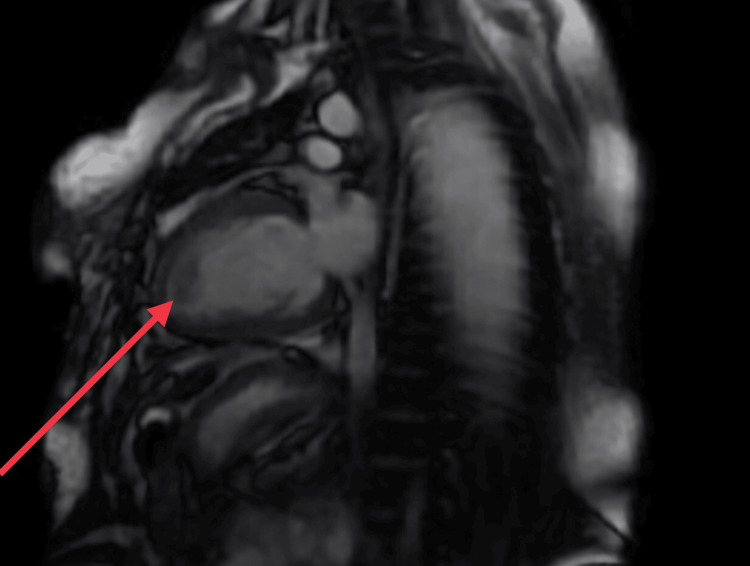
Two-chamber view on cardiac MRI showing apical left ventricular thrombus (arrow)
